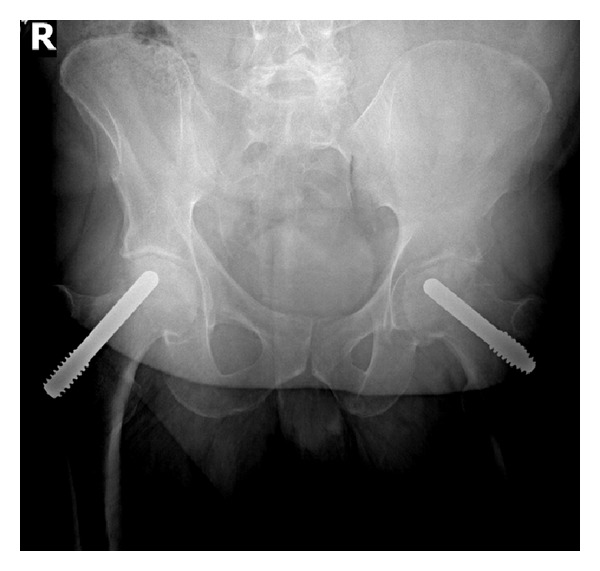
Preoperative pelvis radiograph showing bilateral porous tantalum implants.
